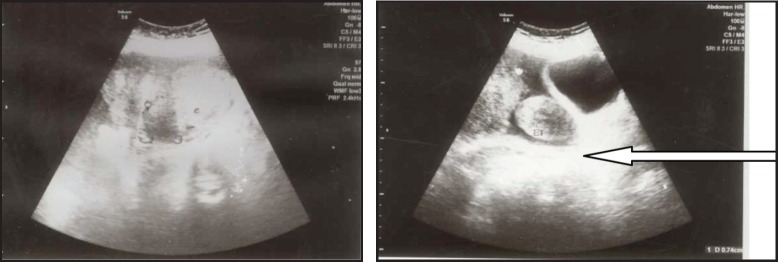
Ultrasonography of whole abdomen and pelvis showed a large mass occupying whole of pelvis just superior to fundus of uterus (not arising from the uterus).
